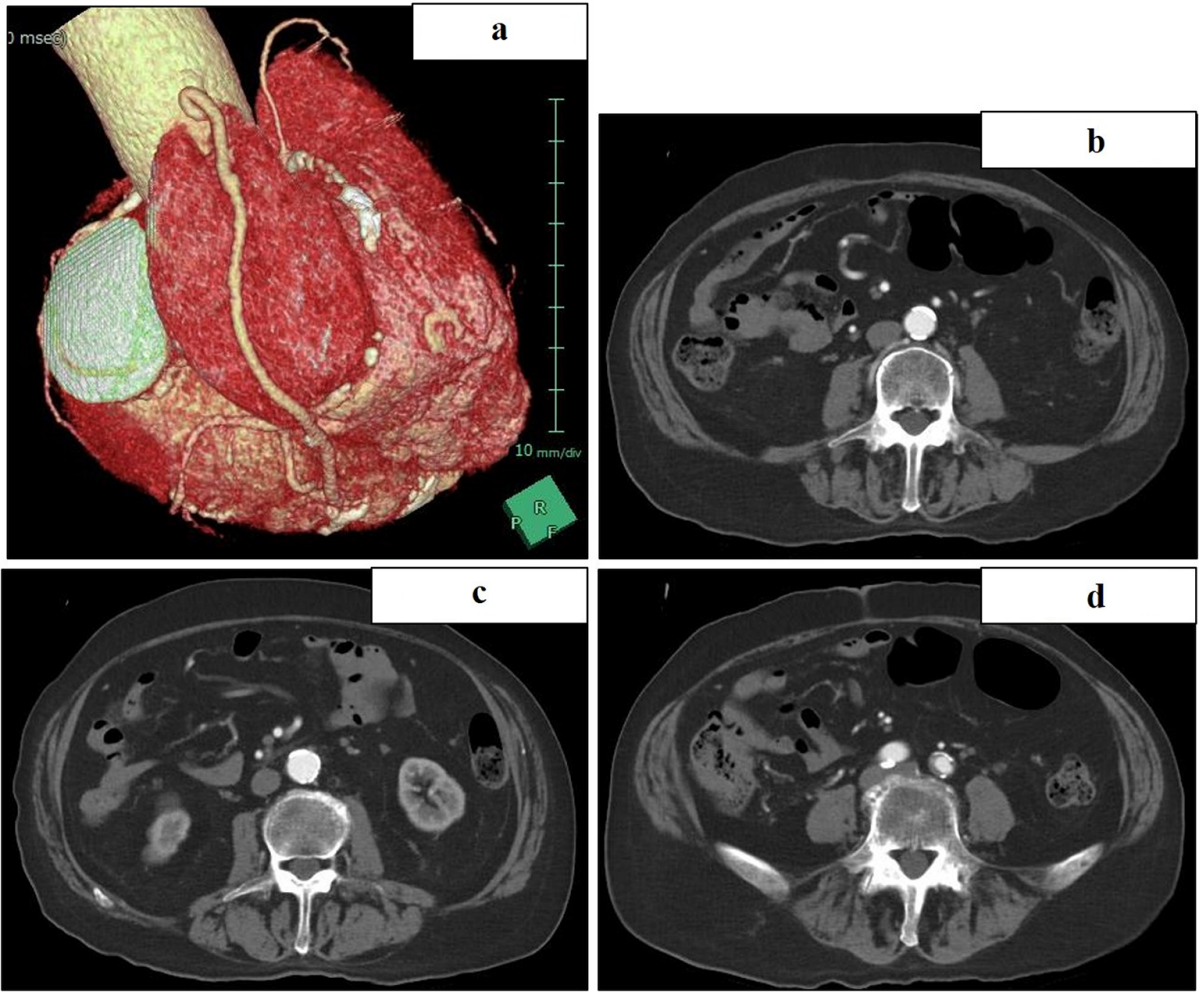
(a) Preoperative 3D CT images. (b–d), preoperative contrast-enhanced CT images from the abdominal aorta extending to the iliac arteries. CT: Computed Tomography.
